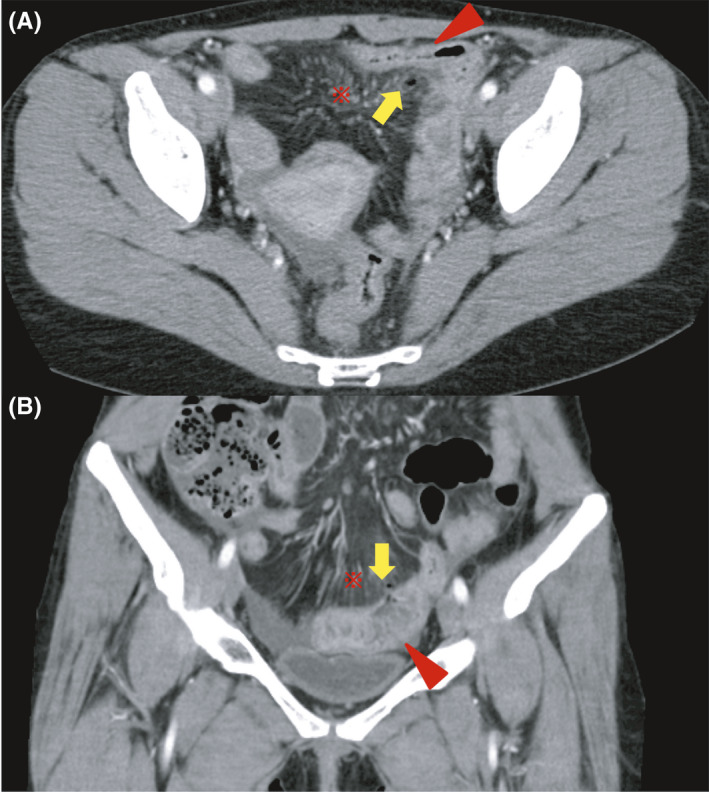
A, B, CT findings showed peritoneal thickening (red asterisk), free air (yellow arrow), and thickening of the small intestine (red arrowhead)
